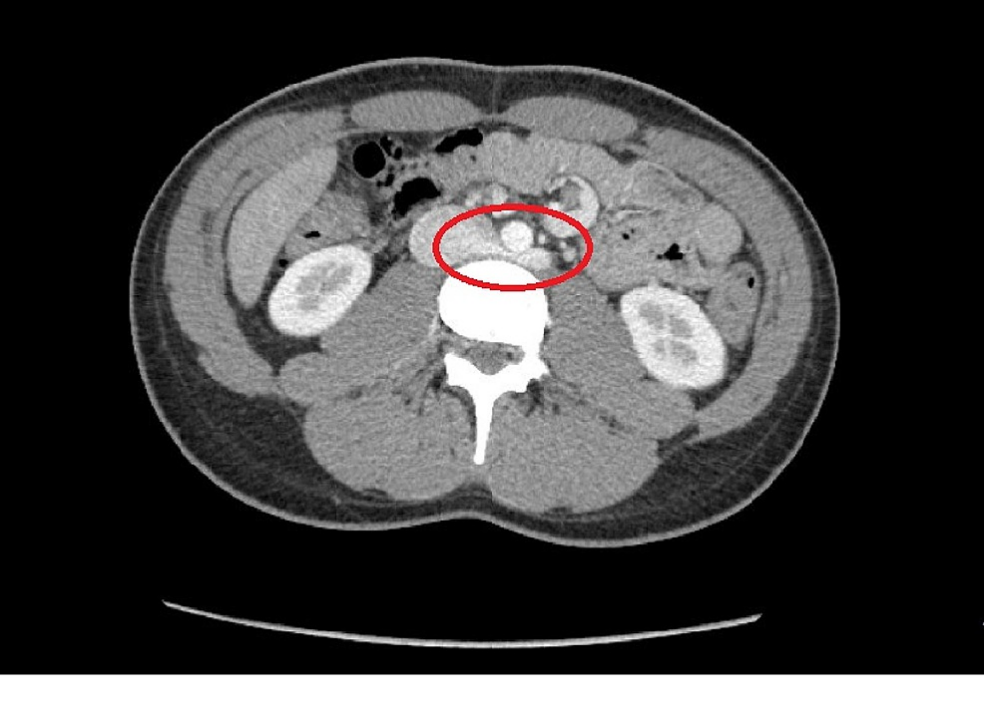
Axial contrast-enhancement CT of the abdomen at the level of the LRV outflow into the inferior vena cava. The red circle shows the abnormal retroarotic course of LRV, causing an anatomic compression between the aorta and the underlying vertebral body suggestive of posterior nutcracker syndrome LRV, left renal vein; IVC, inferior vena cava
